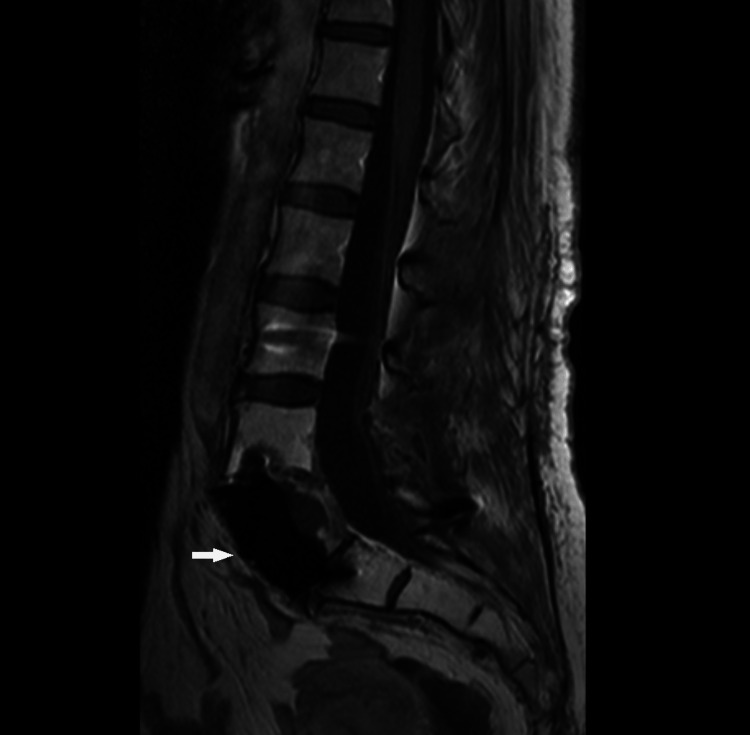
Lumbar MRI after surgery, sagittal enhanced T1WI. Lumbar MRI showed no persistence of disease on sagittal-enhanced T1WI.
